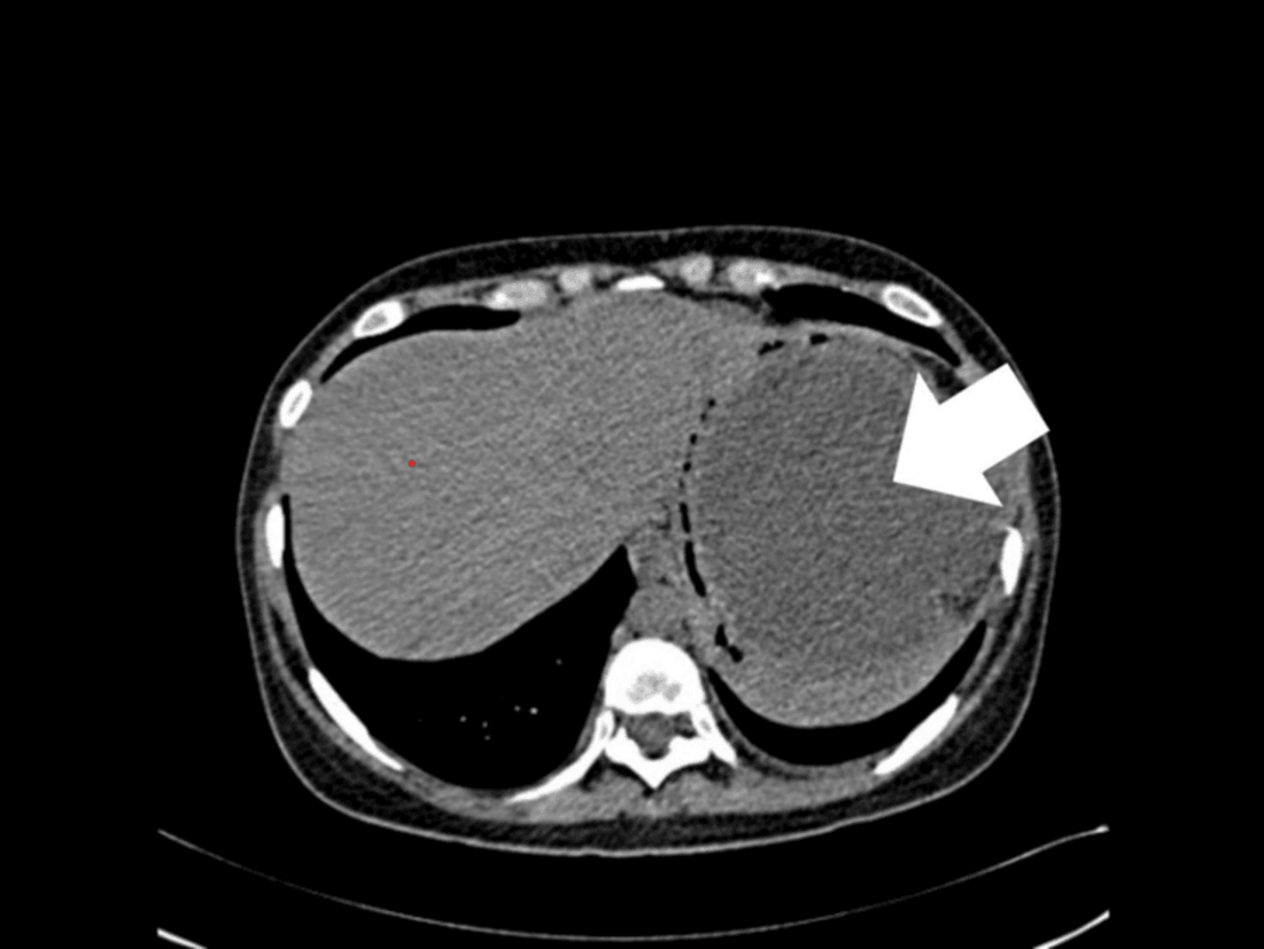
Triphase CT scan of the abdomen - axial view, non-contrast phase The image shows a large pancreatic pseudocyst in the left lateral retroperitoneal location measuring approximately 187 x 85 mm, encasing the distal pancreas with mildly dysmorphic pancreatic parenchyma suggestive of pancreatic pseudocyst CT: computed tomography
